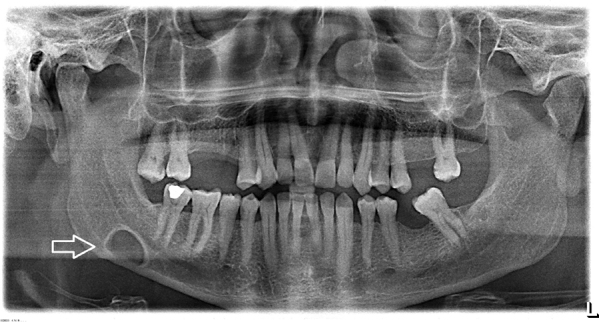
Oval shaped, well-bordered cystic lesion (white arrow) between second molar and the mandibular angle, slightly above the inferior mandibular line in panoramic radiograph.
